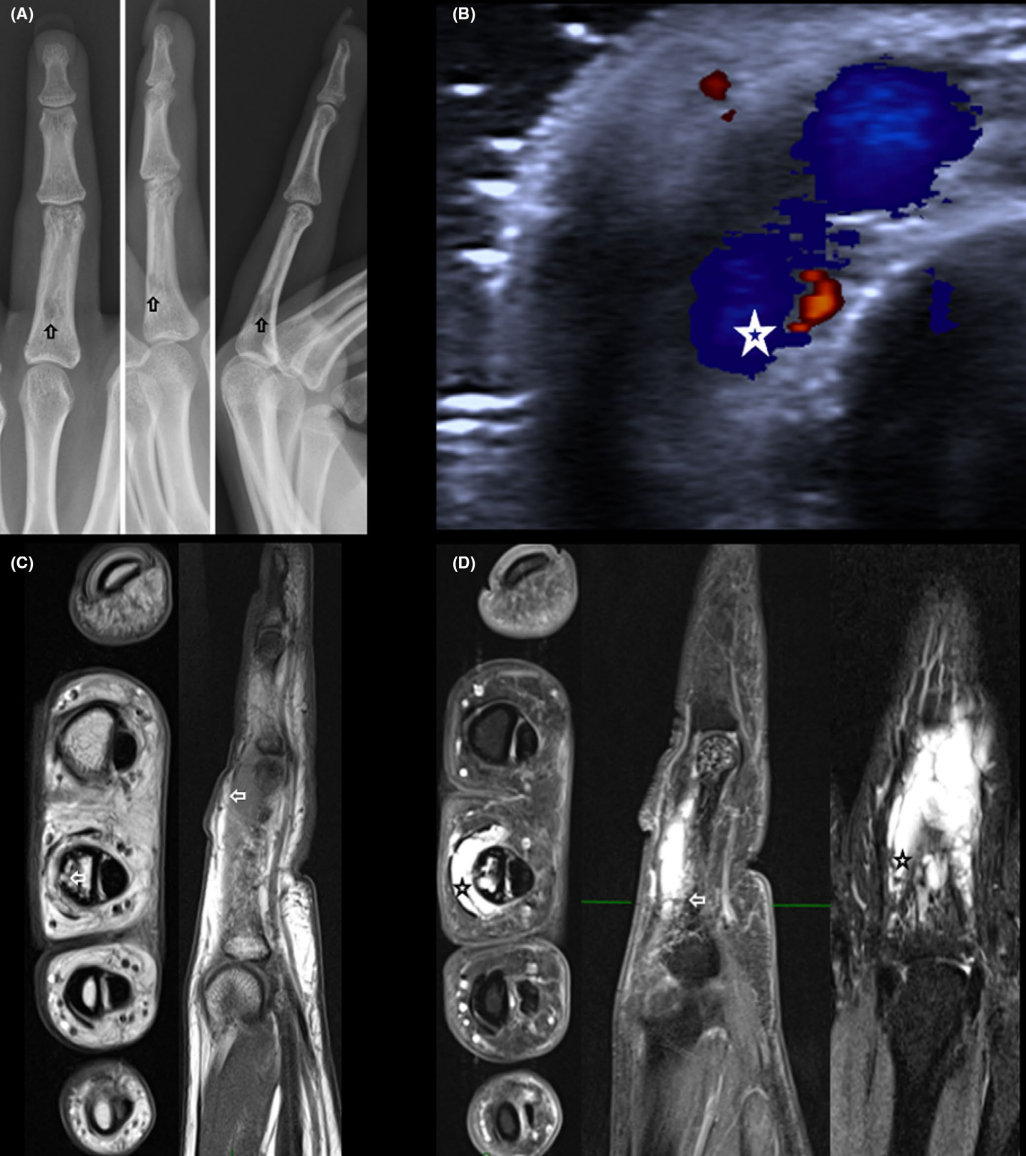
A, Anteroposterior, lateral, and oblique radiographs of the middle finger showing a circular radiolucent image with thinning of the posterior cortical area (
